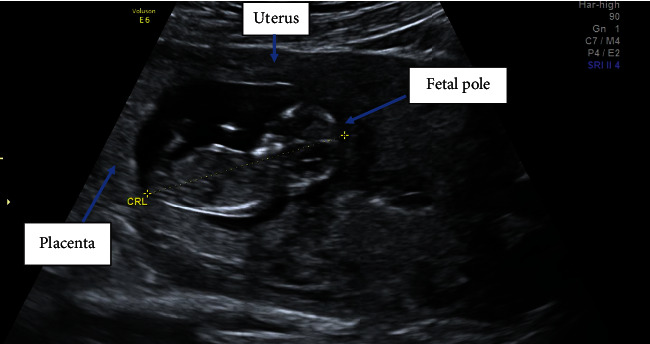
First trimester ultrasound demonstrating a viable intrauterine pregnancy.
